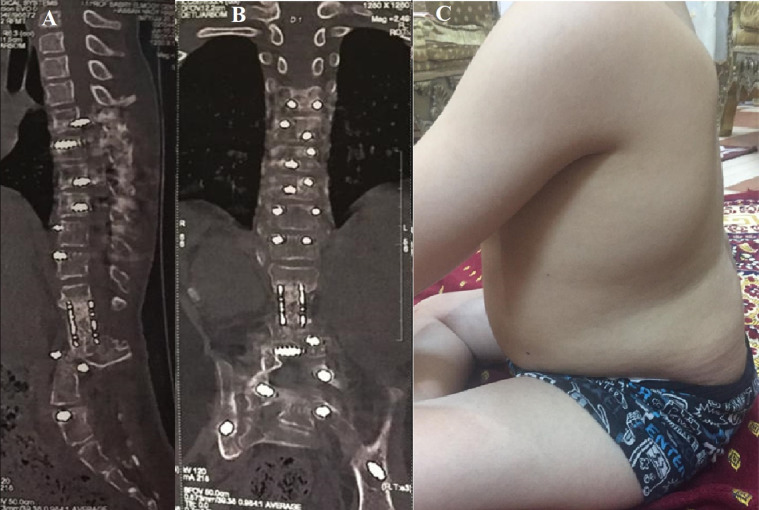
Last follow-up showing continuous bony bar inside cage. (A) Sagittal CT scan. (B) Coronal CT scan. (C) Clinical lateral photo showing sagittal profile.
